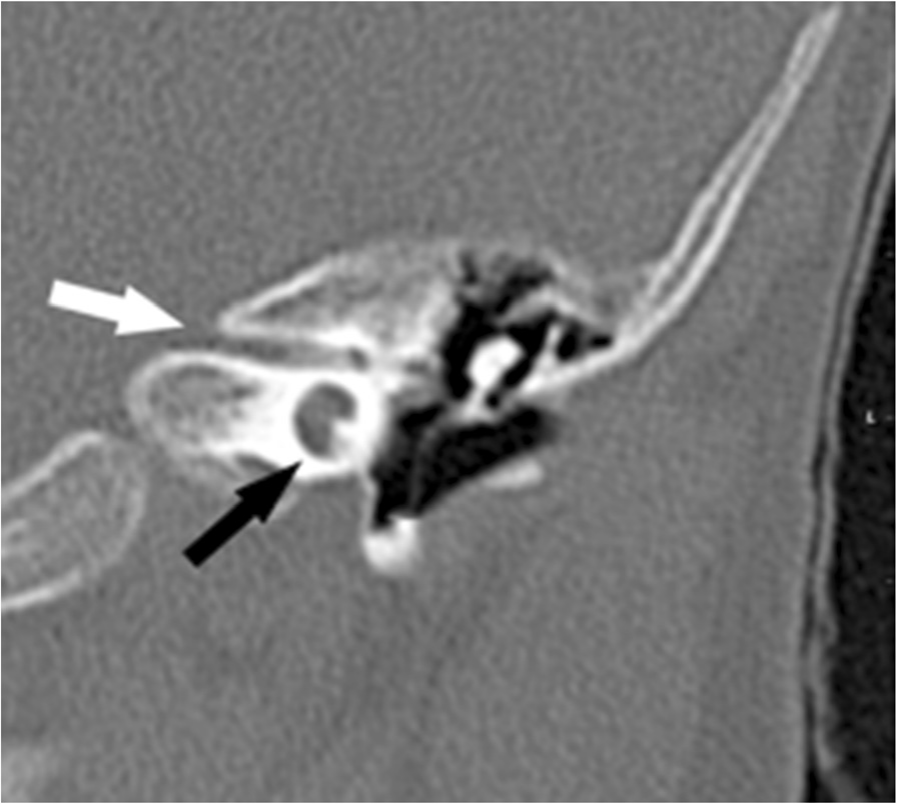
High resolution CT of the left petrosus bone. The coronal view demonstrates the widened single basal cochlear turn (black arrow) and the narrowing of the auditory canal (white arrow). The patient did not show an enlarged vestibular aqueduct (EVA) but similar findings of the right petrosus bone.
